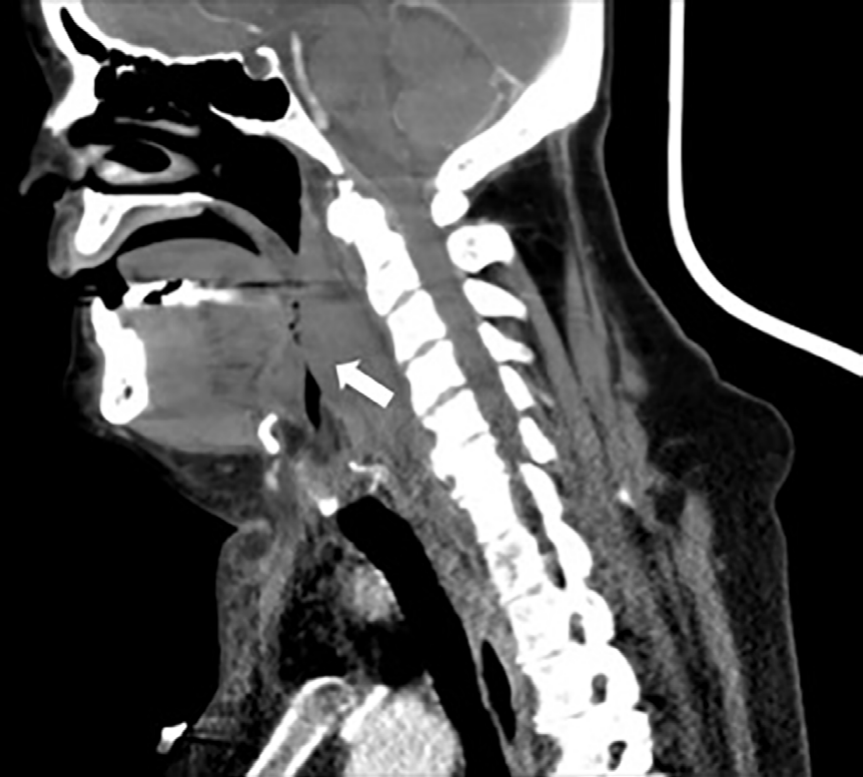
Sagittal view of a non-contrast computed tomography of the neck demonstrating an extensive retropharyngeal hematoma causing mass effect on the airway (arrow).
